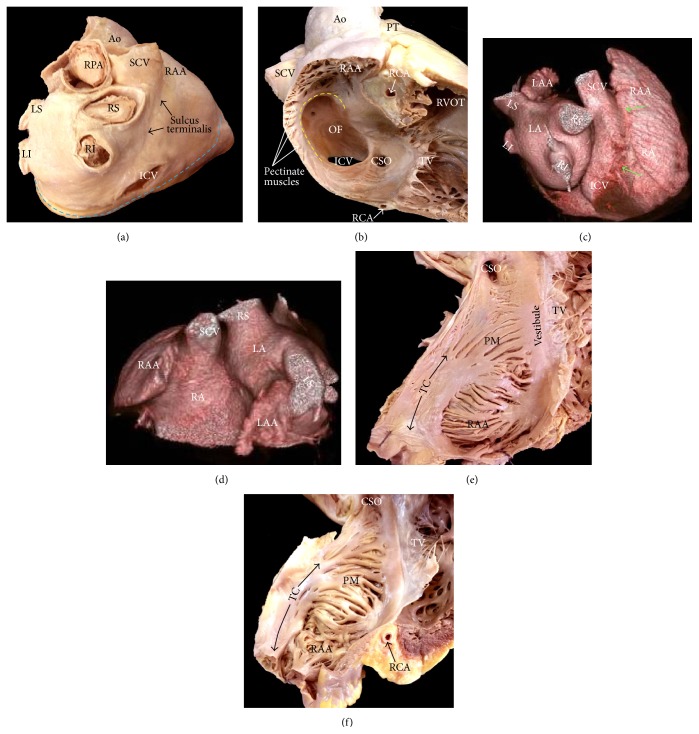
(a) The heart is viewed in attitudinally appropriate position. As can be seen, the right atrium lies anterior to its alleged left-sided counterpart. Note the arrangement of the sulcus terminalis and the atrioventricular or coronary groove (blue broken line). (b) The right atrium is shown in right anterior oblique projection. The terminal crest (yellow broken line) arches anterior to the orifice of the superior caval vein and extends toward the inferior caval vein. (b, c) Spatial relationship of the atrial structures. Right posterior (b) and superior (c) views of volume rendered CT angiographies are shown. The right atrium (RA) body (sinus venarum) is shown extending between the SCV and ICV. The right atrial appendage (RAA) is large with a wide neck compared to the left atrial appendage (LAA). Note prominent pectinate muscles of the RAA. The terminal groove (green arrows) is seen between the RAA and the venous part of the RA. The left atrium (LA) is located superior and posterior to the right atrium. (e, f) Endocardial aspects of the lateral wall of the right atrium opened. Note in (e) that the pectinate muscles have a uniform parallel alignment almost without crossovers between them. In contrast, the pectinate muscles in (f) have a nonuniform arrangement with abundant interlacing trabeculations between them. Ao = aorta, CSO = coronary sinus orifice, ICV = inferior cava vein, LA = left atrium, LAA = left atrial appendage, LI = left inferior pulmonary vein, LS = left superior pulmonary vein, OF = oval fossa, PM = pectinate muscles, PT = pulmonary trunk, RAA = right atrial appendage, RCA = right coronary artery, RI = right inferior pulmonary vein, RPA = right pulmonary artery, RS = right superior pulmonary vein, RVOT = right ventricle outflow tract, SCV = superior cava vein, TC = terminal crest, and TV = tricuspid valve.
